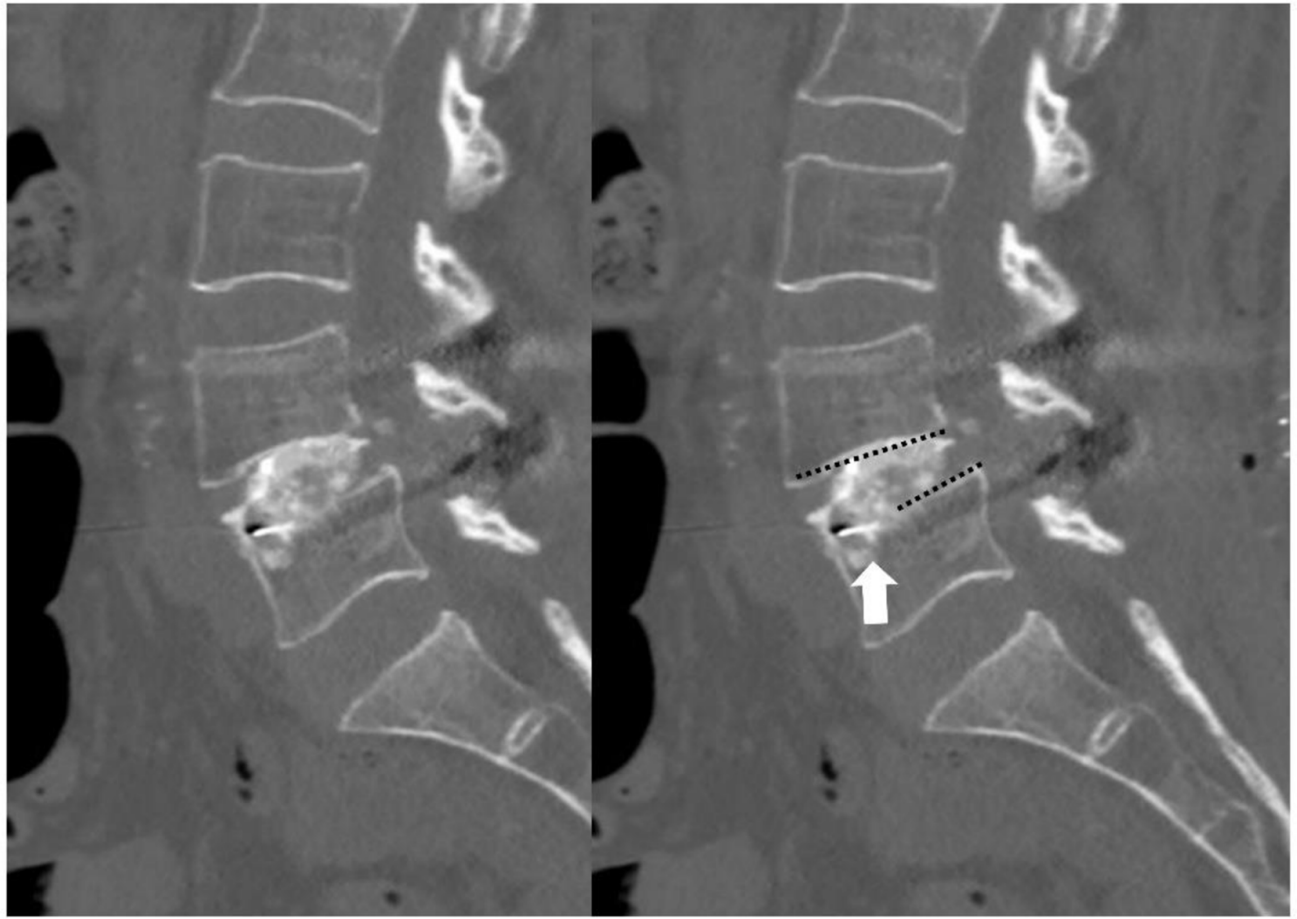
Intraoperative endplate injury on 1mm thin cut reconstructed sagittal scan of postoperative CT, black dotted line - endplate, white arrow - intraoperative endplate injury
